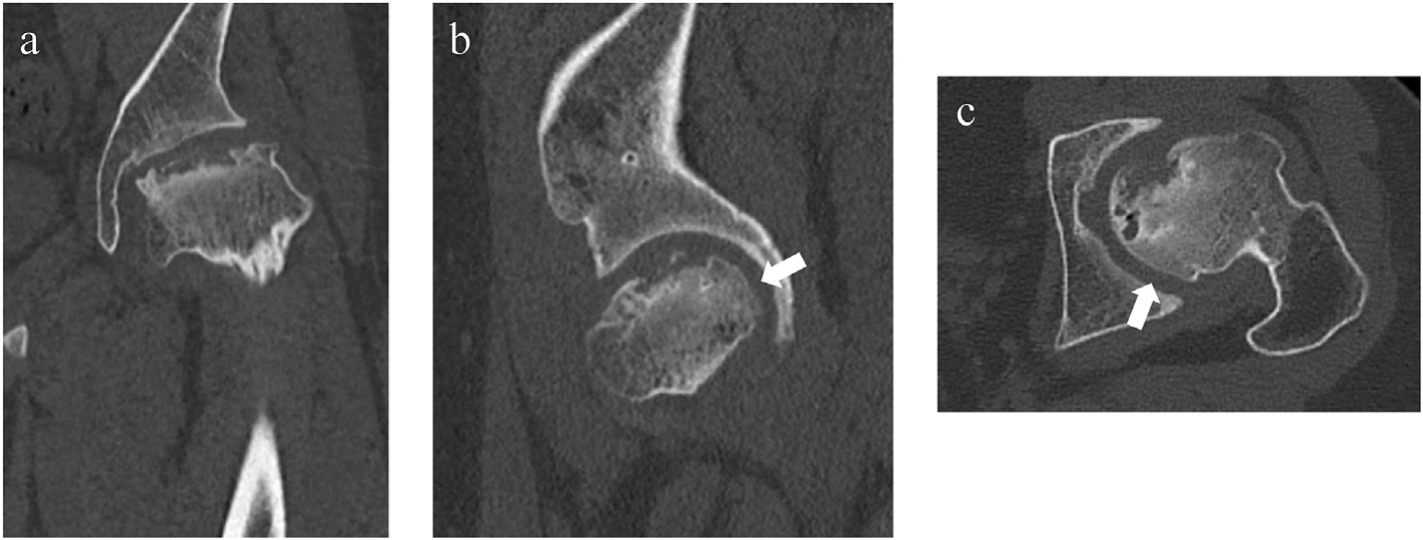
Computed tomography, a) Coronal view, b) Sagittal view, c) Axial view.
